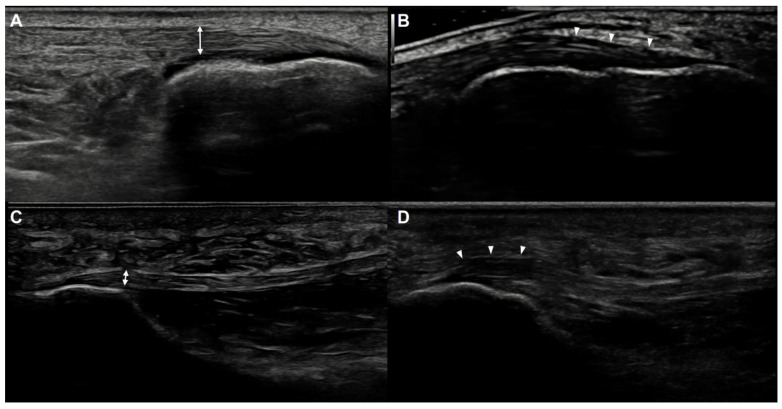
Ultrasonographic measurement of the thickness of the Achilles tendon and plantar fascia. (A) The maximal thickness of the insertional area of the Achilles tendon (two-sided arrow) was measured within 2 cm of the calcaneal insertion, where the tendon is attached to the bone. (B) A hypoechoic area and loss of the fibrillar pattern (arrowheads) were evident at the insertional area of the Achilles tendon. Note the toggling technique to keep the direction of the beam as close to perpendicular as possible in relation to the insertional area of the Achilles tendon was used. (C) The maximal thickness of the plantar fascia (two-sided arrow) was measured within 1 cm of the calcaneal attachment. (D) A hypoechoic area and loss of the fibrillar pattern (arrowheads) were evident at the attachment site of the plantar fascia.
